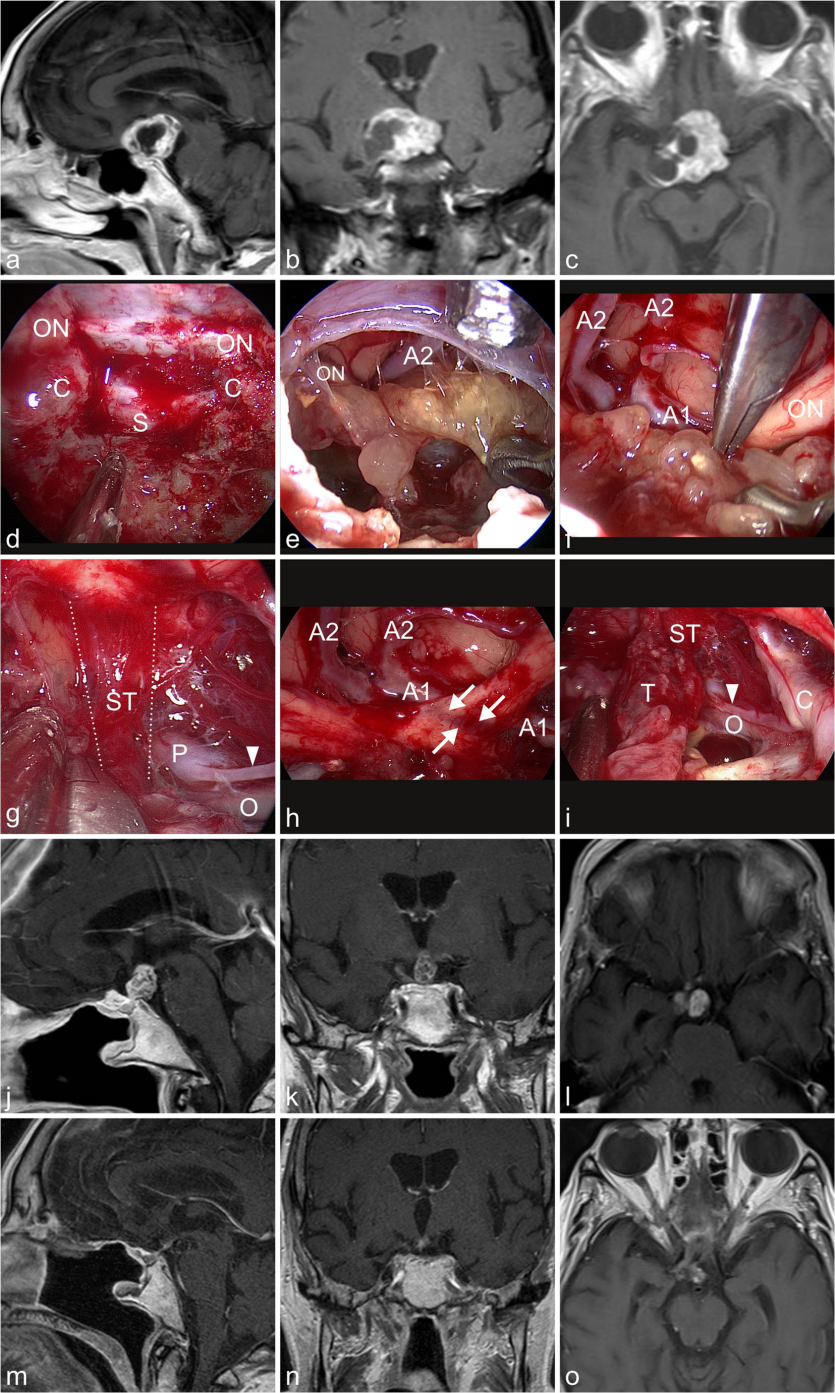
The 84-year-old lady presented with visual problems. The ophthalmological examination revealed a bilateral loss of visual acuity and bitemporal visual field deficits. The endocrinological evaluation demonstrated an intact pituitary function. a–c MR imaging revealed a suprasellar partially solid, partially cystic contrast enhancing lesion which was highly suspicious of a craniopharyngioma. d A transtuberculum-transplanum approach was performed. The protuberances of the optic nerves (ON), clinoidal carotid arteries (C), and sellar dura (S) are seen. e After debulking of the tumor, the superior surface of the tumor was exposed under view of a 30° endoscope. The A2 segment of the right anterior cerebral artery and the right optic nerve (ON) are seen. f The tumor was dissected from the optic chiasm using the bimanual traction-countertraction technique with two forceps (optic nerve (ON), A1- and A2-segments of the anterior cerebral arteries (A1, A2). g At the dorsal tumor surface, the infiltrated pituitary stalk (ST, in between the dashed line) is visualized. Oculomotor (O), P1-segment of the posterior cerebral artery (P), and posterior communicating artery (arrowhead) are seen. h After tumor resection severe grooving (arrows) within the left optic nerve caused by the tumor pressure against the nerve and the A1-segment of the anterior cerebral artery (A1 und A2) is seen. i The final inspection shows the tumor remnant (T) at the infiltrated pituitary stalk (ST), the posterior communicating artery (arrow head), the supraclinoidal carotid artery (C), and the oculomotor nerve (O). j–l MR imaging obtained 3 months after surgery showed the tumor residual at the pituitary stalk. m–o MR imaging obtained 22 months after fractionated stereotactic radiotherapy with 45 Gy revealed an impressive shrinking of the tumor. The patient is doing well under replacement therapy of hydrocortisone and thyroxine. The ophthalmological examination revealed a marked improvement of the visual field deficits
